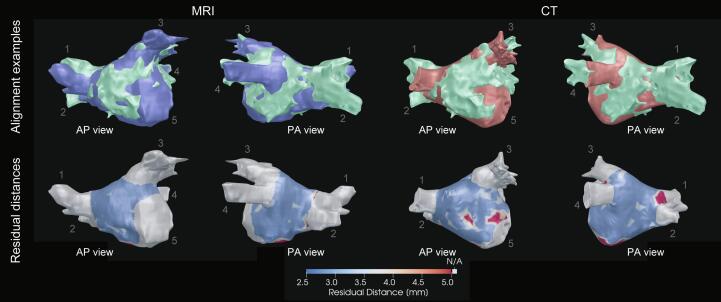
Alignment examples The top row shows anterior-poster (AP) and posterior-anterior (PA) views of alignment examples of one patient’s MRI (blue, left) and CT anatomy (right, red) with the corresponding EAM anatomy (green). The bottom row shows the regional residual Euclidean distances between the aligned anatomies (bottom). 1, Right superior PV; 2, Right inferior PV; 3, Left superior PV; 4, Left inferior PV; 5, Mitral valve anulus; MRI, magnetic resonance imaging; CT, computed tomography; EAM, electro-anatomical mapping. (For interpretation of the references to colour in this figure legend, the reader is referred to the web version of this article.)
